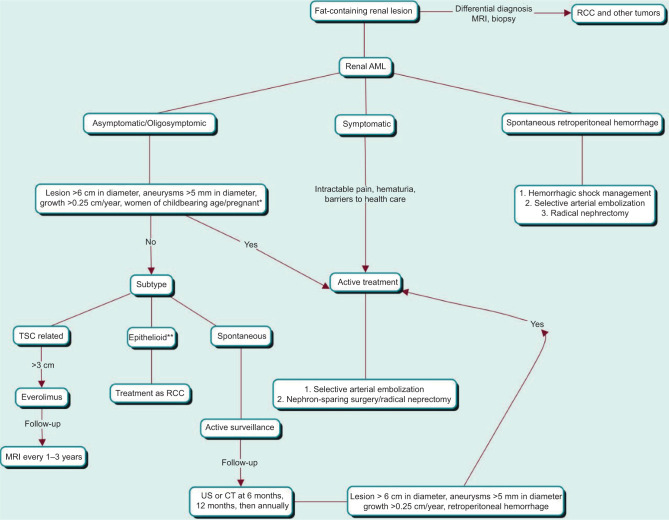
Proposed updated management algorithm. RCC – renal cell carcinoma; AML – angiomyolipoma; TSC – tuberous sclerosis complex; MRI – magnetic resonance imaging; US – ultrasound; CT – computerized tomography. *Associated with other risk factors, according to medical criteria. See characteristics and risk factors in “epithelioid AML.”
